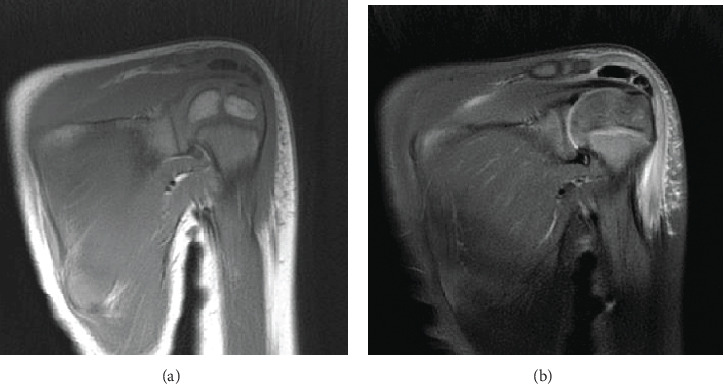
Coronal magnetic resonance imaging of the left shoulder. (a) T1-weighted; (b) T2-weighted fat suppression.
